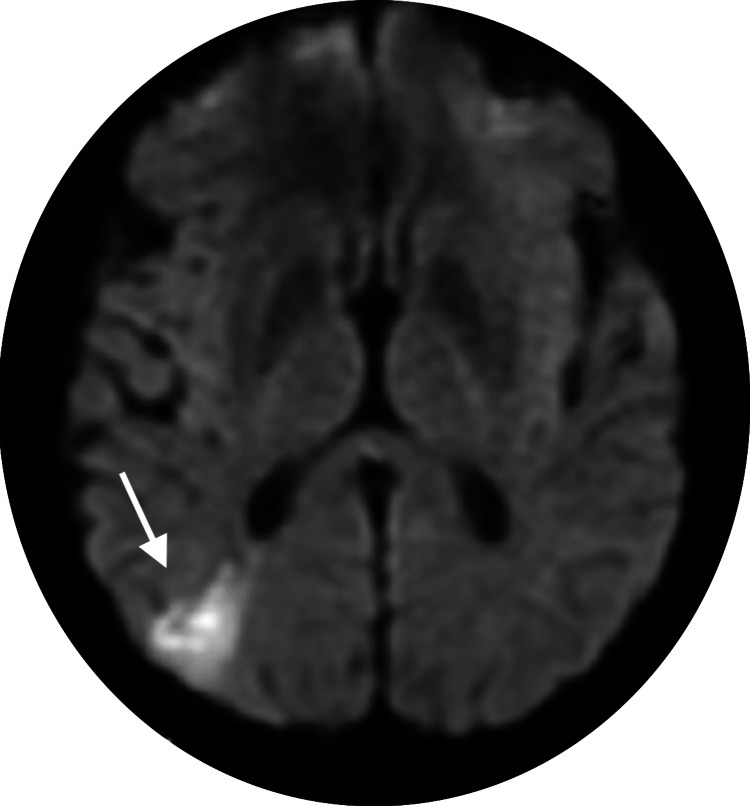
Focal wedge-shaped area of diffusion restriction in right occipital lobe indicating acute infarction on axial DWI DWI: diffusion-weighted imaging
